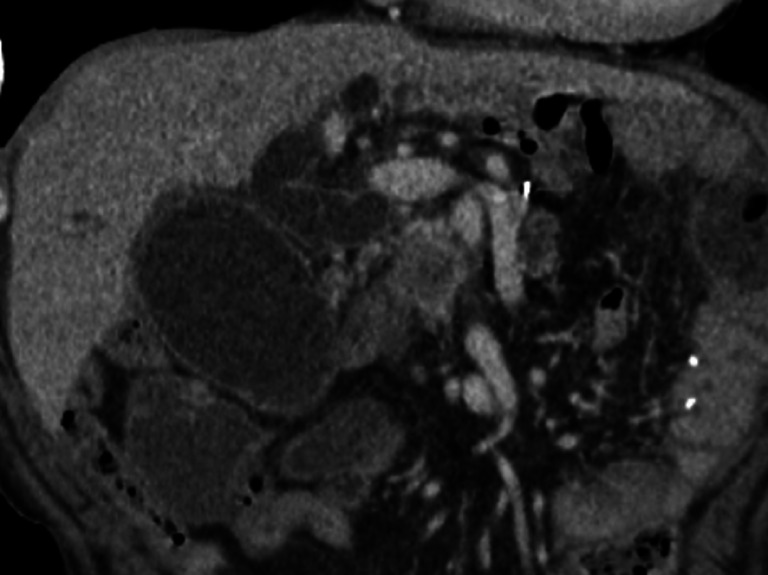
Contrast-enhanced computed tomography (CT) image in coronal section. CT reveals a hypodense mass with a hyperdense rim around the pancreatic head.
